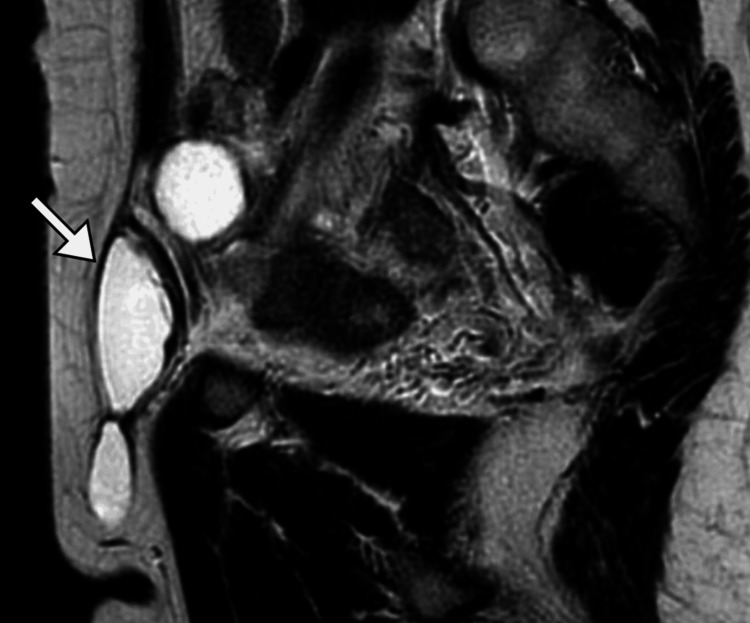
MRI image of the pelvis Selected sagittal MRI image of the pelvis showed a lesion with fluid signal intensity (arrow) in the right inguinal canal. MRI: magnetic resonance imaging
